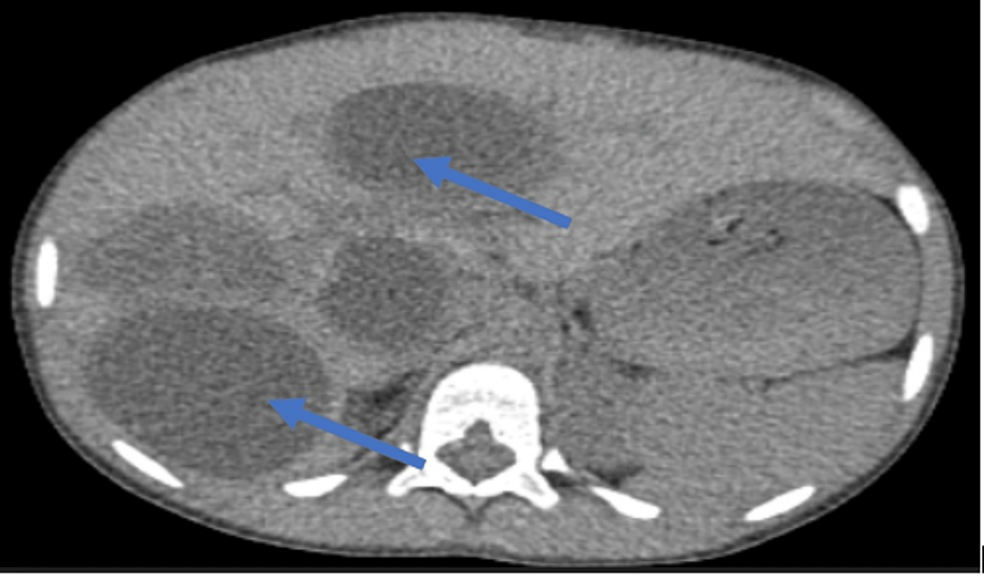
CT scan showing the axial image of multiple hydatid cysts in the liver (arrows).
